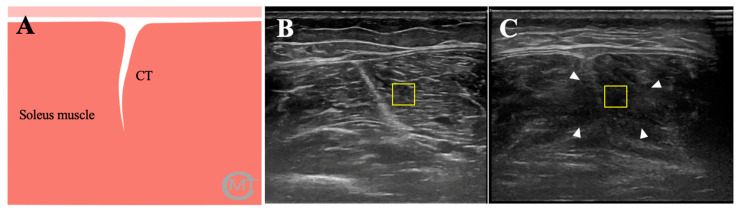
Ultrasound images of central tendon of soleus muscle. (A). Graphic representation of the ultrasound image of the soleus muscle; (B). Ultrasound imaging of health athlete; (C). Ultrasound imaging of an athlete with soleus muscle injury. Ultrasound imaging of patients group shows muscle fiber disorganization at the level of tear (arrowhead). CT, central tendon; ROI.
